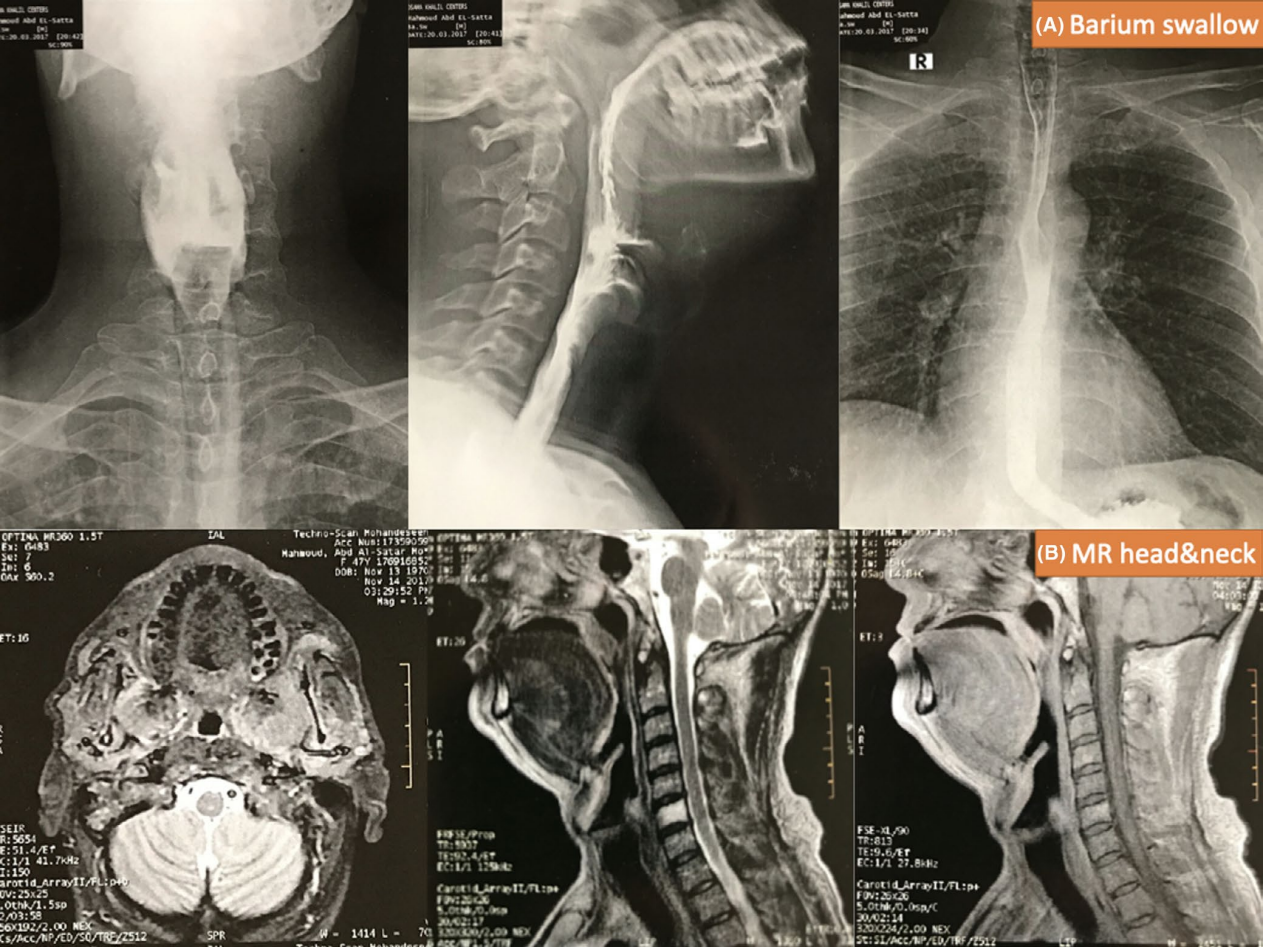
Radiological investigations of the patient; A, Barium swallow and B, MRI of the head and neck region
